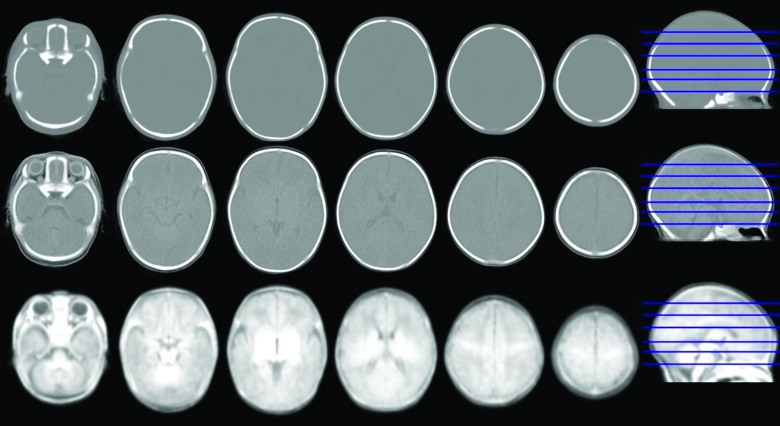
Multi-slice visualization of the proposed neonatal MR-CT head template. The first and second row show some axial slices in Hounsfield units and the intensity-transformed scale of GRAMFC_CT_39-42_, respectively, and the third row shows the corresponding slices from the GRAMFC_T_39-42_ MR template.
